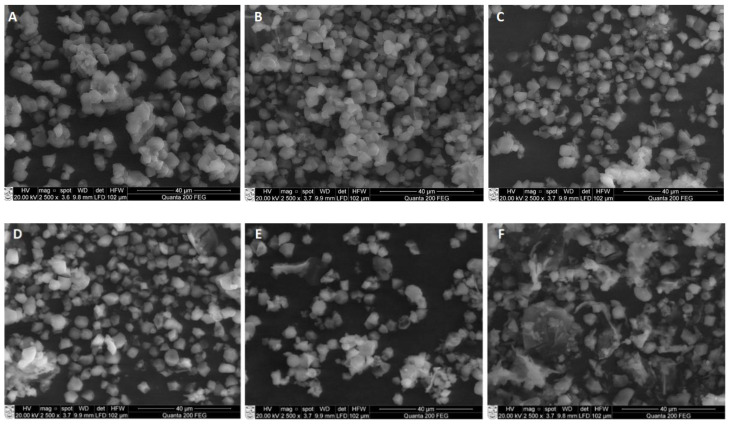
SEM images of native rice starch (A), resistant starch isolated from RB untreated (B) and ultrasonicated (1.8 W/cm^2^, 20 min) at 40 °C (C), 50 °C (D), 60 °C (E), and 70 °C (F) temperatures (magnification ×2500).
